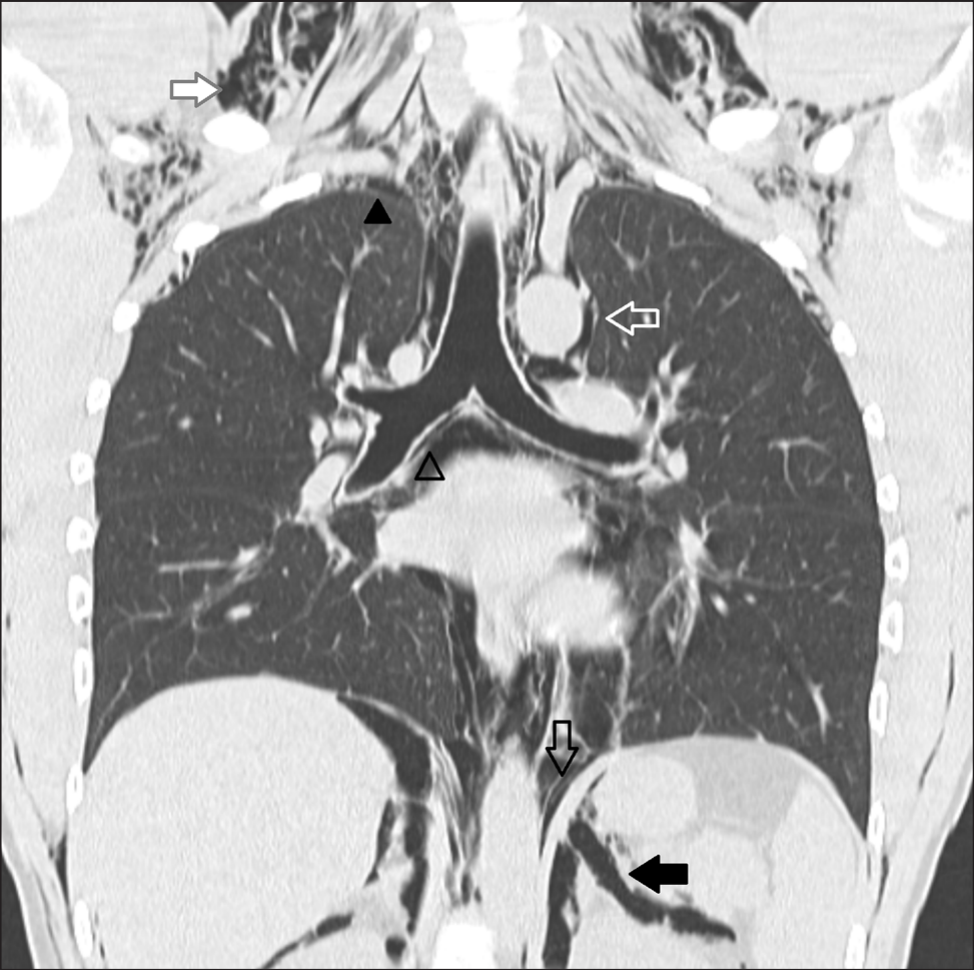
CT of the chest confirming a pneumomediastinum (hollow black arrowhead, hollow black arrow and hollow white arrow) associated with diffuse subcutaneous emphysema (solid white arrow), pneumo(retro)peritoneum (solid black arrow) and pneumothorax (solid black arrowhead).
